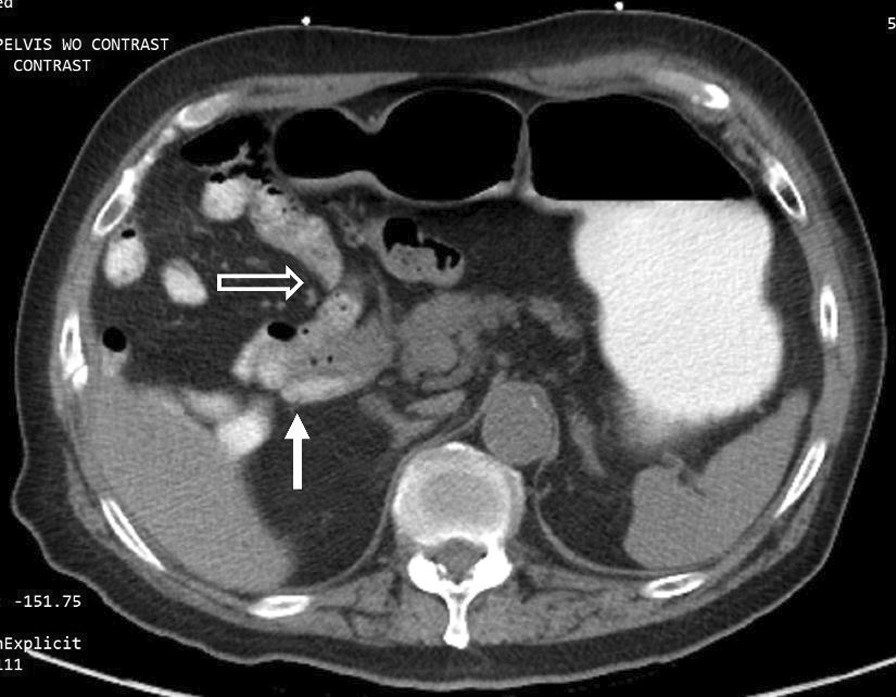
Location of D3. CT of the abdomen without intravenous contrast and with positive oral contrast. The duodenum (hollow arrow) fails to pass under the superior mesenteric artery to the left upper quadrant. The duodenojejunal flexure (solid arrow) is abnormally located in the right upper quadrant
